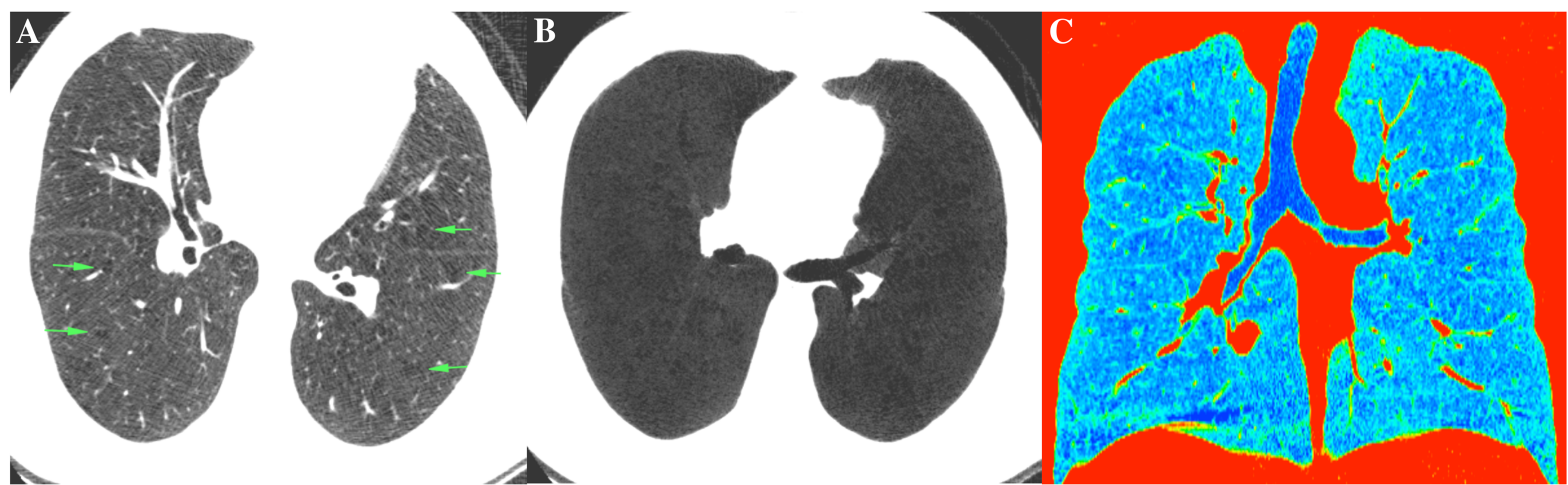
CT scan of a patient with FEV_1_/FVC = 73.4, but a total low-attenuation area of 18%. (A) Axial CT image demonstrating areas of emphysematous changes (arrowheads). (B) Axial MINIP reconstruction of the same patient shows a predominantly diffuse pattern of low-attenuation (C) Colored reconstruction of a coronal image demonstrating multiple low-attenuation areas in blue.
